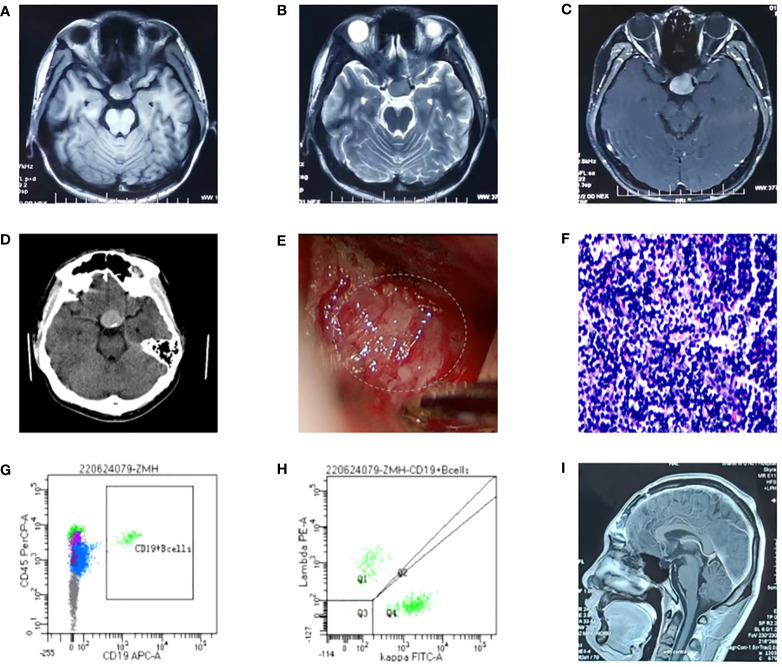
(A-C) Head MRI: the sella was expanded, with an oval slightly long T1 and long T2 abnormal signal, and there was uneven enhancement and a clear boundary. The neurohypophysis is clearly shown, the left optic chiasm is compressed, and the internal carotid artery in the left cavernous sinus is partially surrounded. The intracranial segment of the left optic nerve were compressed and slightly thinned, and the enhanced scan showed mild enhancement; (D) Head CT: the sella is enlarged, high-density nodules are seen in the pituitary fossa, the size is about 2.1*2.8cm, and the CT value is about 56HU; (E) The tumor was found to have abundant blood supply during the operation; (F) Pathological section: Proliferating tumor cells and have a starry sky appearance and they are dense, with medium cell volume, thin cytoplasm, large and slightly irregular nuclei, and fine chromatin; (G, H) Flow cytometry, and we found that B lymphocytes with CD19+CD20+ accounted for about 19.8% of lymphocytes, with polyclonal expression of Kappa and Lambda; (I) Postoperative re-examination of head MRI showed no tumor recurrence.
